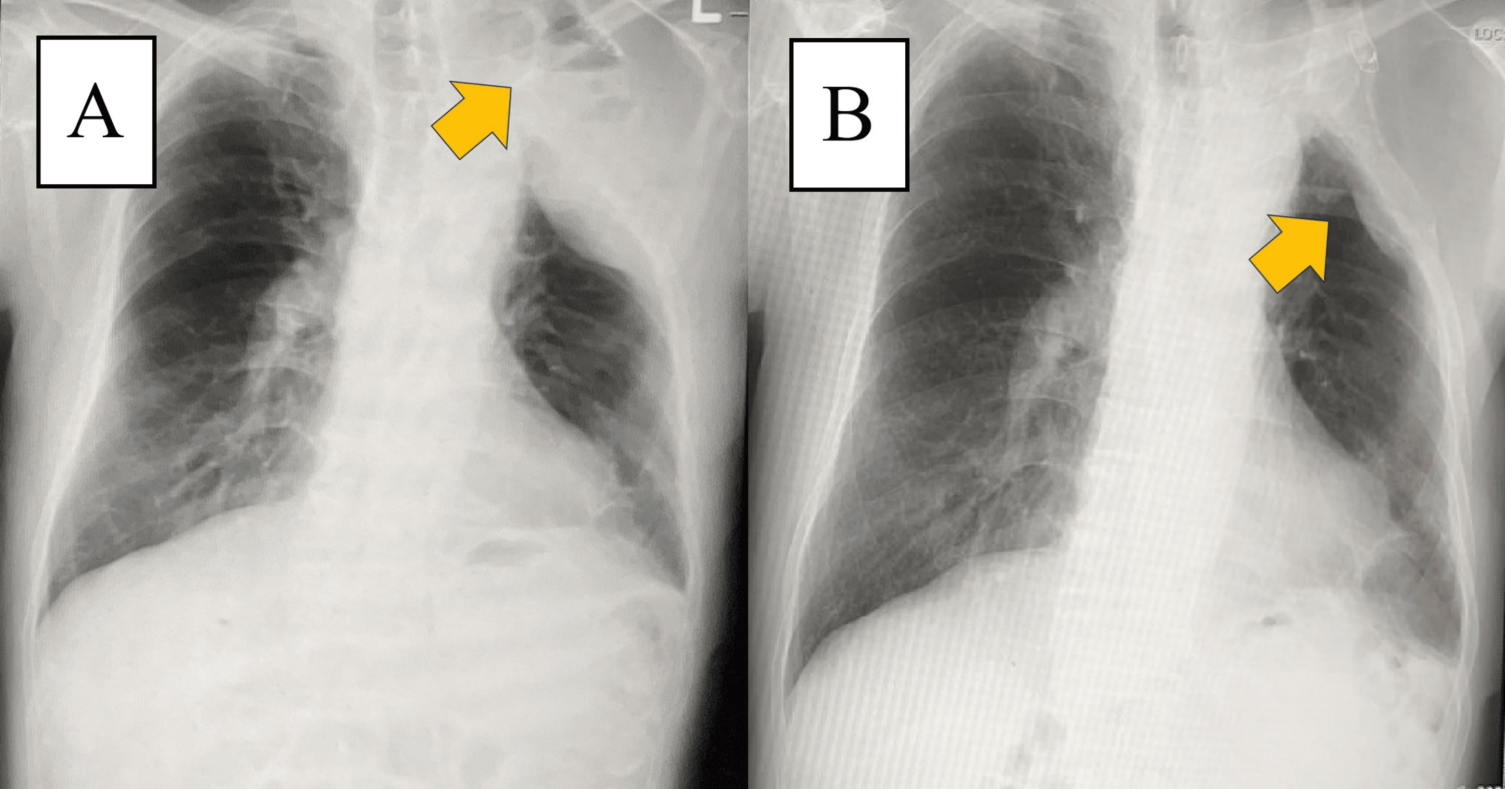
Postoperative chest X-ray findings (A) On the chest X-ray one month after surgery, a pleural effusion and an air-fluid level were observed in the left upper lung field (arrow). (B) On the chest X-ray two years after surgery, the pleural effusion decreased (arrow).
